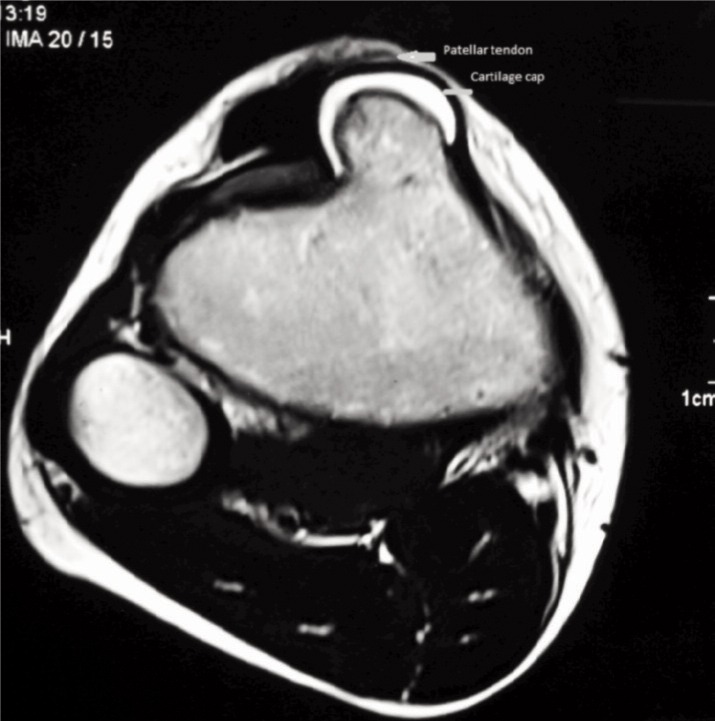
Fat-saturated T2 axial section of magnetic resonance imaging shows a well-defined osseous protrusion from the anteromedial aspect of tibial metaphysis underneath the patellar tendon.
